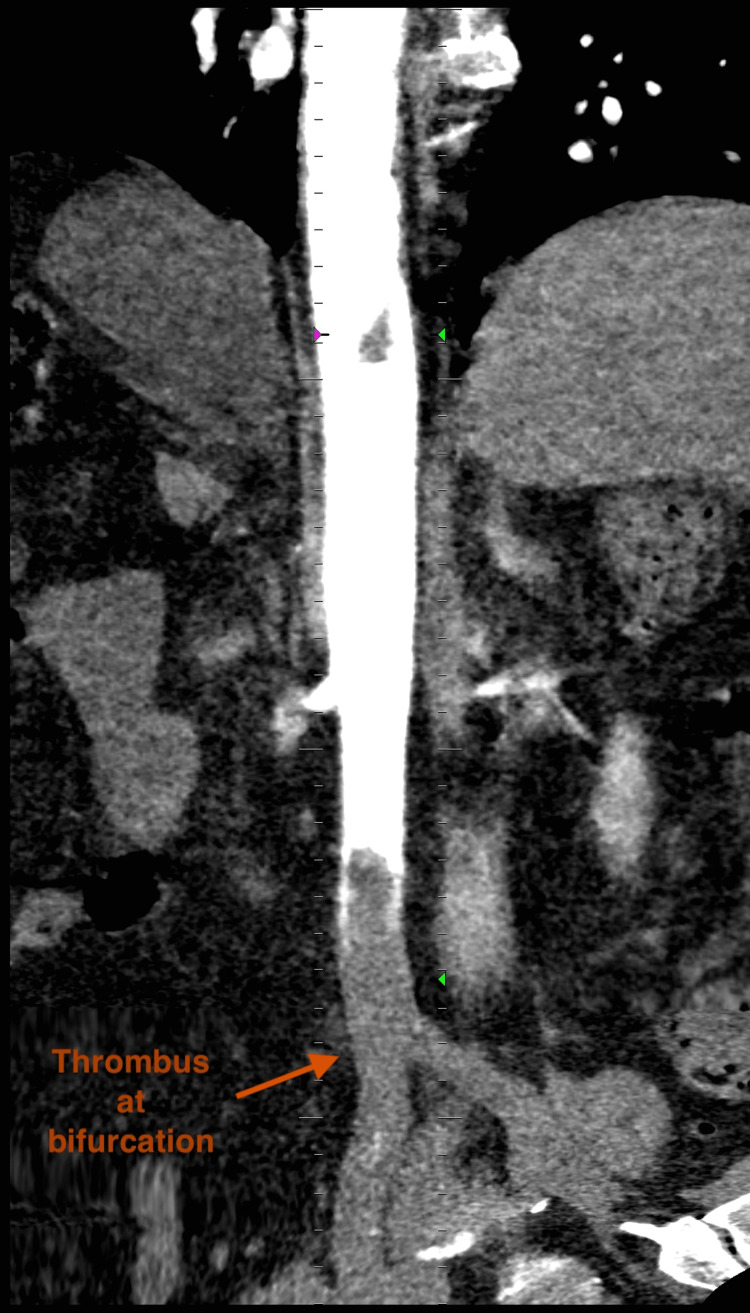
CT Angiogram - Coronal Plane
